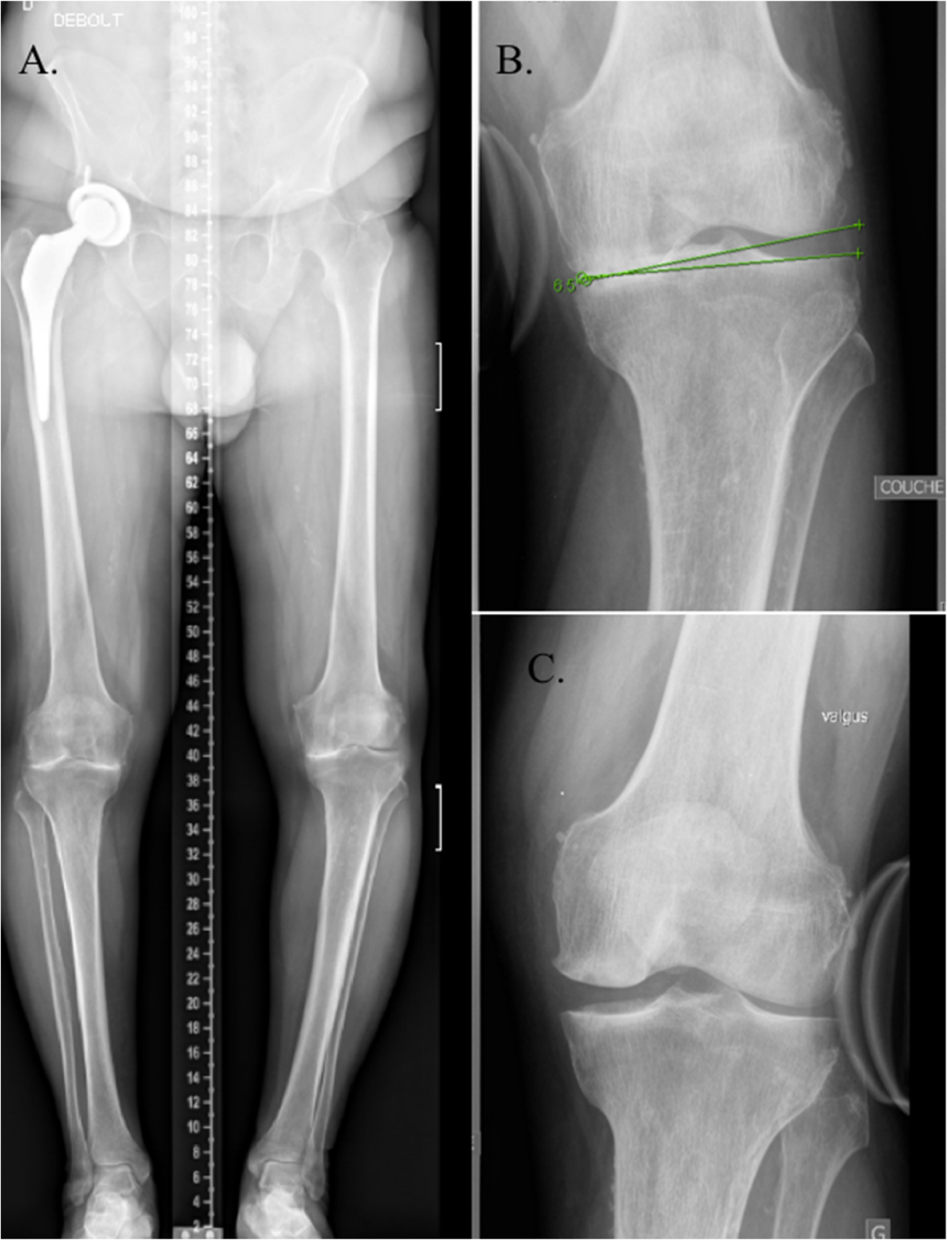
Preoperative X-rays showing soft tissue imbalance. a. Patient with a preoperative advanced osteoarthritis and assessment of soft tissue imbalance before osteotomy b. Preoperative JLCA under varus stress c. Preoperative JLCA under valgus stress
